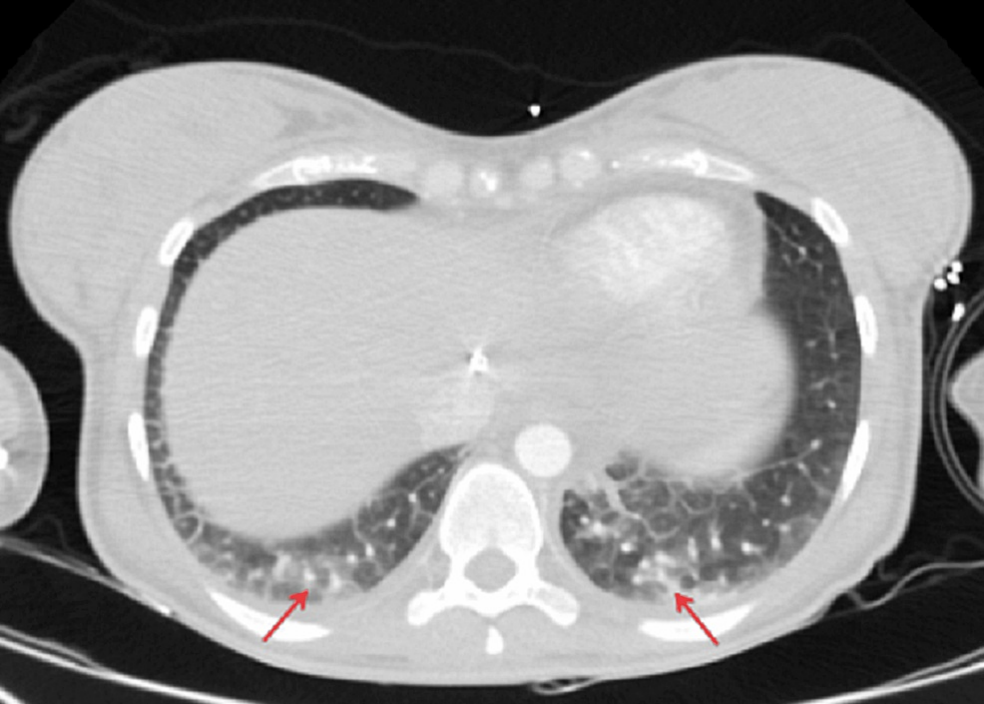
Axial CT image of the thorax with lung algorithm demonstrates multiple poorly defined centrilobular nodules in the lower lobes, mainly on the left side with a tree-in-bud appearance (red arrows).
